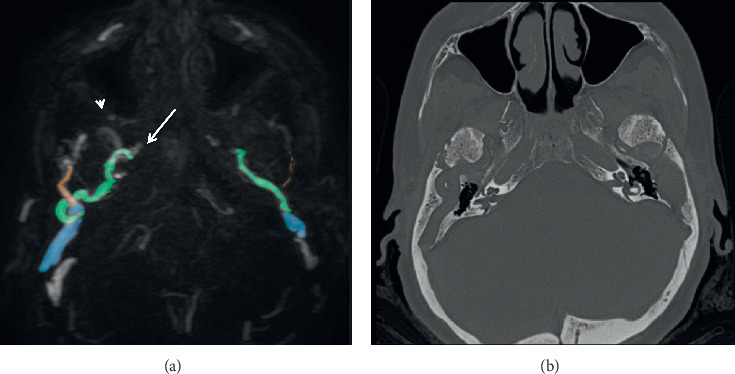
Axial MRV (a) and HRCT mIP (b) showing striking asymmetry in the petrosquamosal sinuses (PSS) and canals, bilaterally (blue); middle meningeal sinus (green) draining into the cavernous sinus (arrow) and pterygoid venous plexus (arrow head) via the foramen ovale; retromandibular vein (orange) draining into external jugular vein; mastoid emissary vein (white) posterior to PSS.
